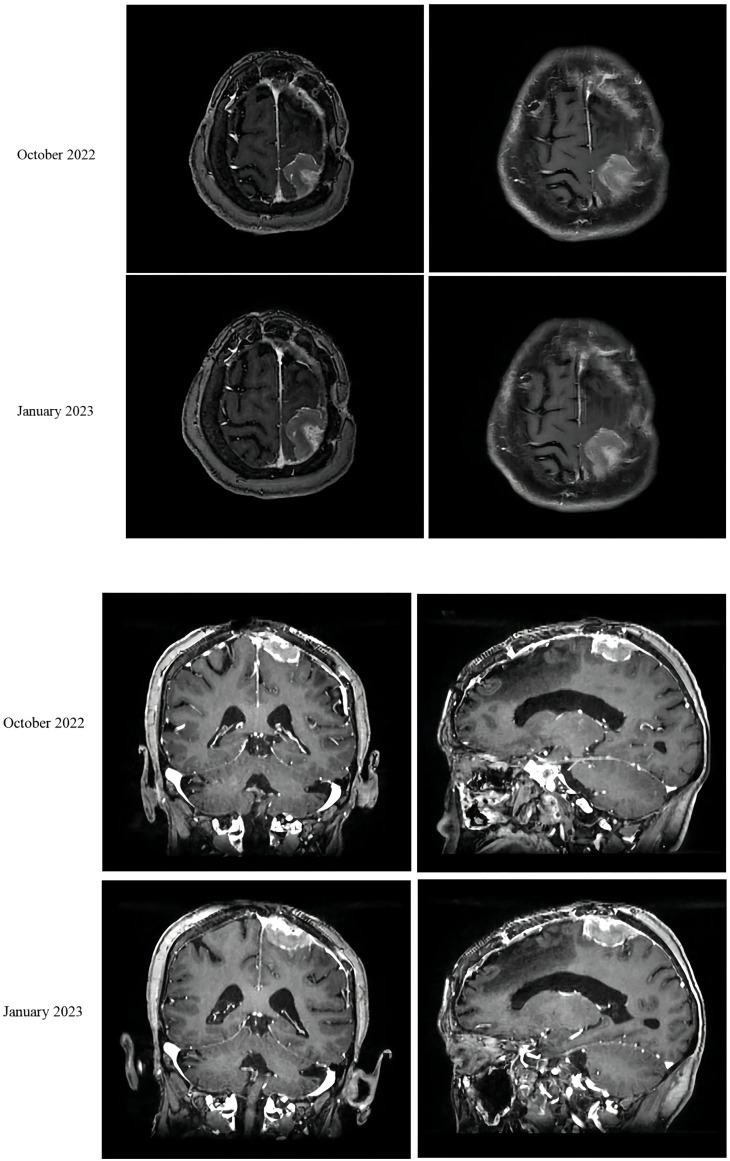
MRI of the patient’s meningioma in October 2022 and January 2023.
